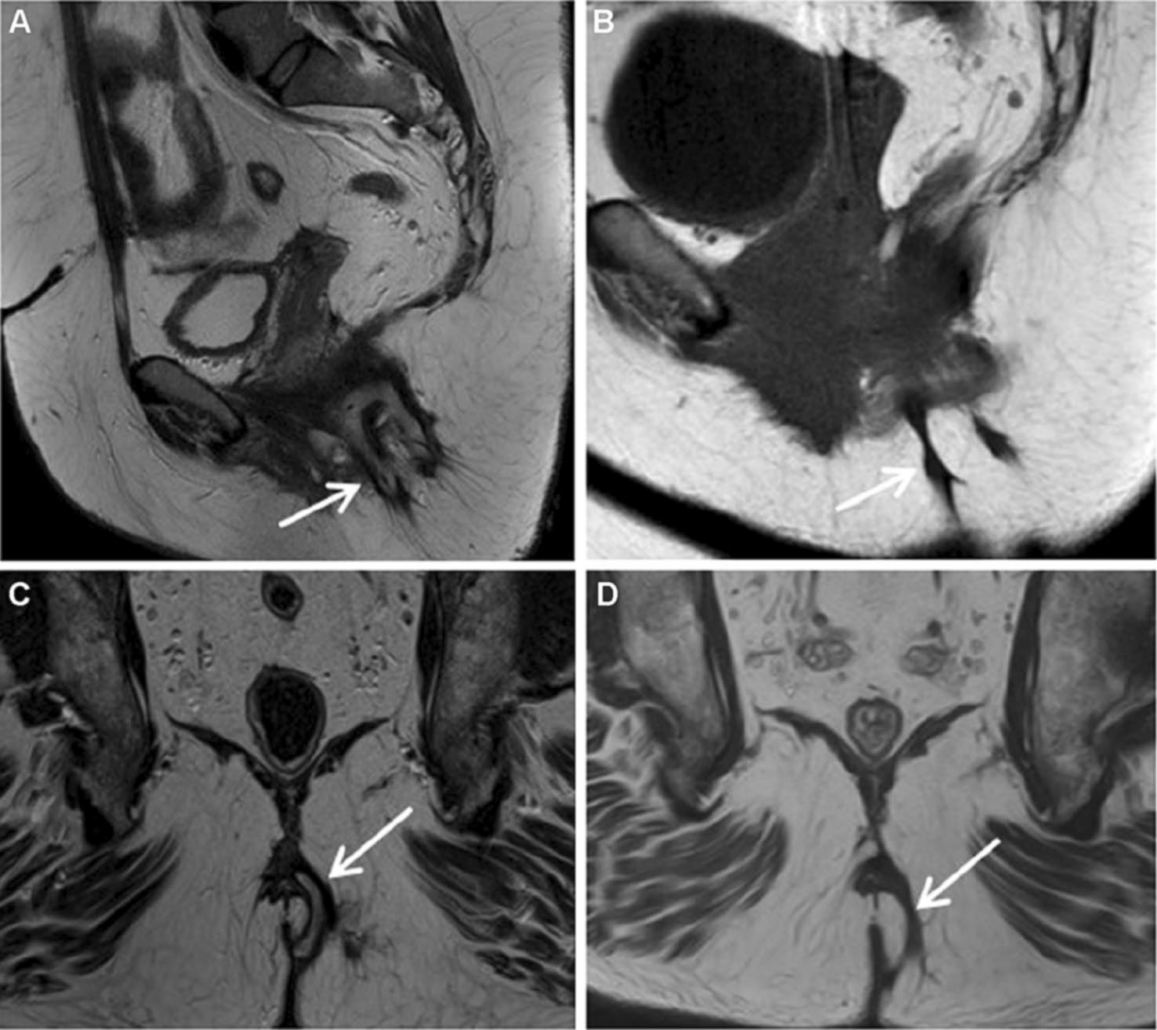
Preoperative and postoperative MRI in 2 patients with fistula healing, showing: A preoperative MRI (sagittal view) of a transsphincteric fistula with horseshoe extension complete obliteration of the fistula tract (patient 1); B postoperative MRI (sagittal view) at 6 months shows complete obliteration of the fistula tract with fibrotic tissue (patient 1); C preoperative MRI (coronal view) of a transsphincteric fistula (patient 2); D postoperative MRI (coronal view) at 6 months shows complete obliteration of the fistula tract with fibrotic tissue (patient 2). *MRI* magnetic resonance imaging
